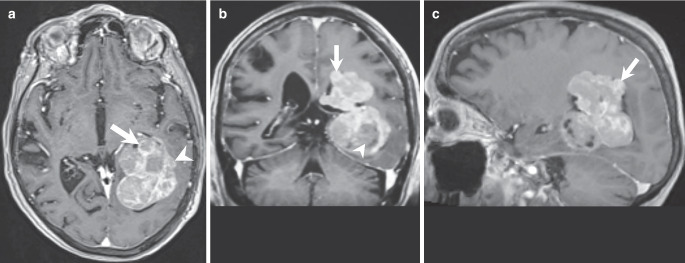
Axial (a), coronal (b), and sagittal (c) T1-weighted images acquired after gadolinium (Gd) administration show marked contrast enhancement of the lesion (arrows). Areas of the lesion without contrast enhancement, indicative of regressive tumour changes, are noted (b, arrowhead). Additionally, invasion into the surrounding brain parenchyma is evident at the lateral margin of the tumour (a, arrowhead)
